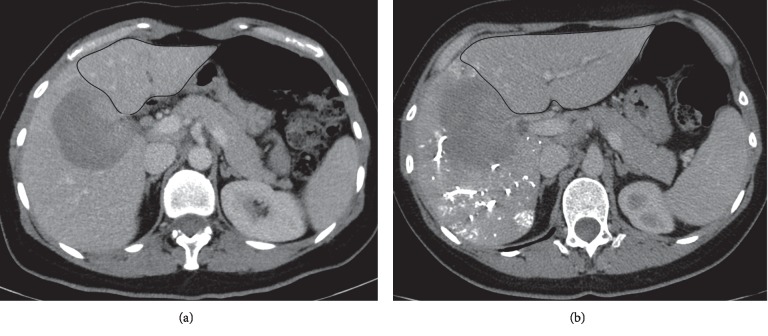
(a) Computed tomography in the portal venous phase acquired before portal vein embolization shows a small left liver (future liver remnant circled in black line). (b) Computed tomography in the portal venous phase shows a significant increase in the left liver (future liver remnant circled in black line) 28 days after portal vein embolization with NBCA.
